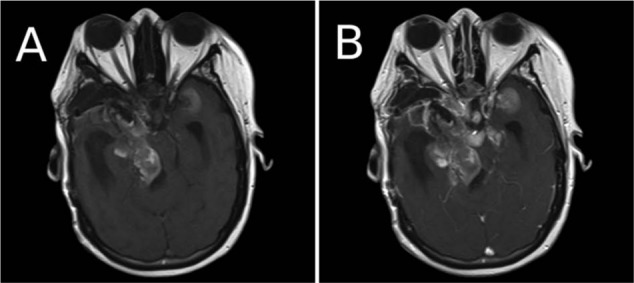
Final magnetic resonance image after the third surgery at our institution. (A) Precontrast T1 image with bright signal typical of central nervous system melanoma. (B) Postcontrast magnetic resonance image showing extensive tumor spread.
